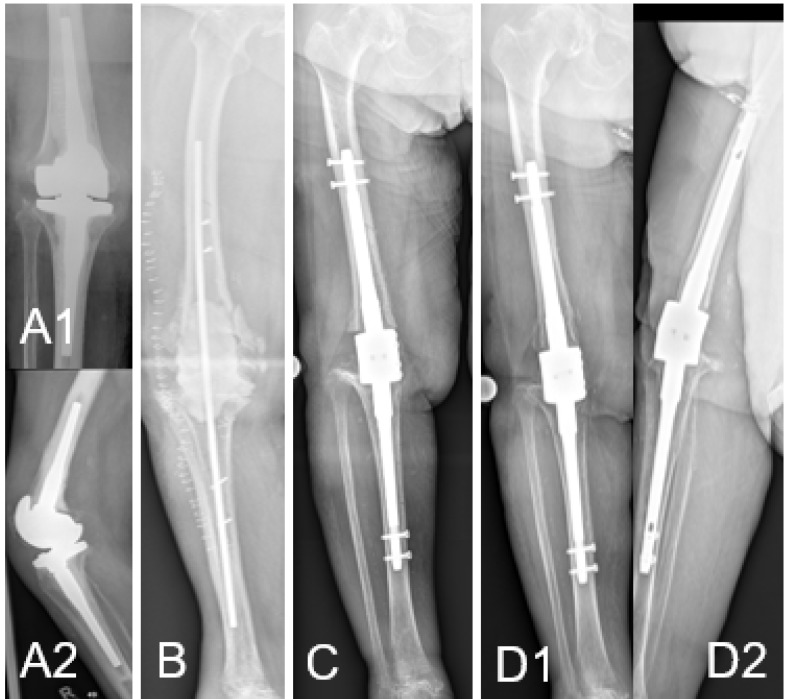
(A) Pre-operative X-ray with knee revision arthroplasty with cemented femoral and tibial stem anchorage without any obvious signs of loosening or osteolysis (A1: ap, A2: lateral). (B) After removal of the revision prosthesis intramedullary rods are placed in the femur and tibia with an antibiotic-loaded PMMA spacer to fill the large dead space as interim knee arthrodesis solution. Cortical windows were needed for complete bone cement removal, which were then fixed with screws. (C) Postoperative ap-view after modular knee arthrodesis with metallic silver coating and connection of the intramedullary femoral and tibial components with a spacer and locking bolts. Intramedullar rods were fixed cementless. (D) 26 months control after modular knee arthrodesis no implant loosening could be evidenced on both ap (D1) and lateral (D2) view.
